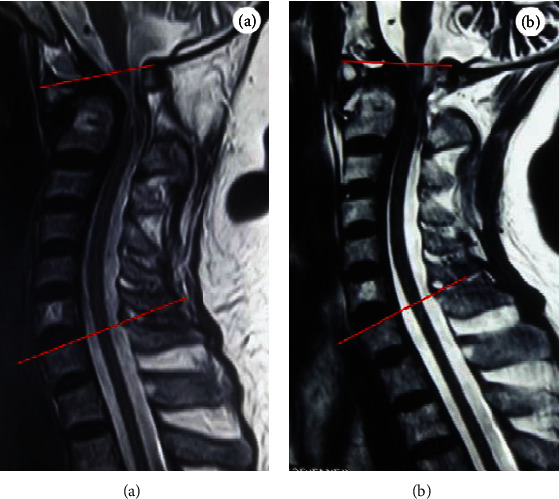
C1-7 Cobb angle is defined as the angle between the superior line of the C1 anterior arch and posterior arch and the C7 superior end plate: (a) the C1-7 Cobb angle on median sagittal neutral MRI; (b) the C1-7 Cobb angle on median sagittal extension MRI. The preoperative sagittal mobility of the cervical spine is defined as angle b − angle a.
